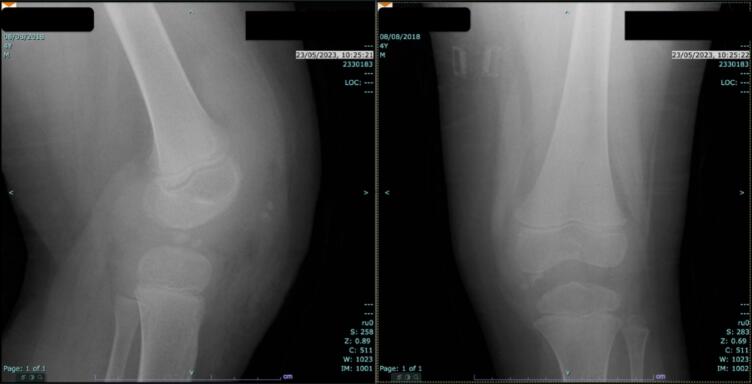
Plain radiographs of the left knee on initial presentation (Left: Lateral view; Right: Anteroposterior view).
